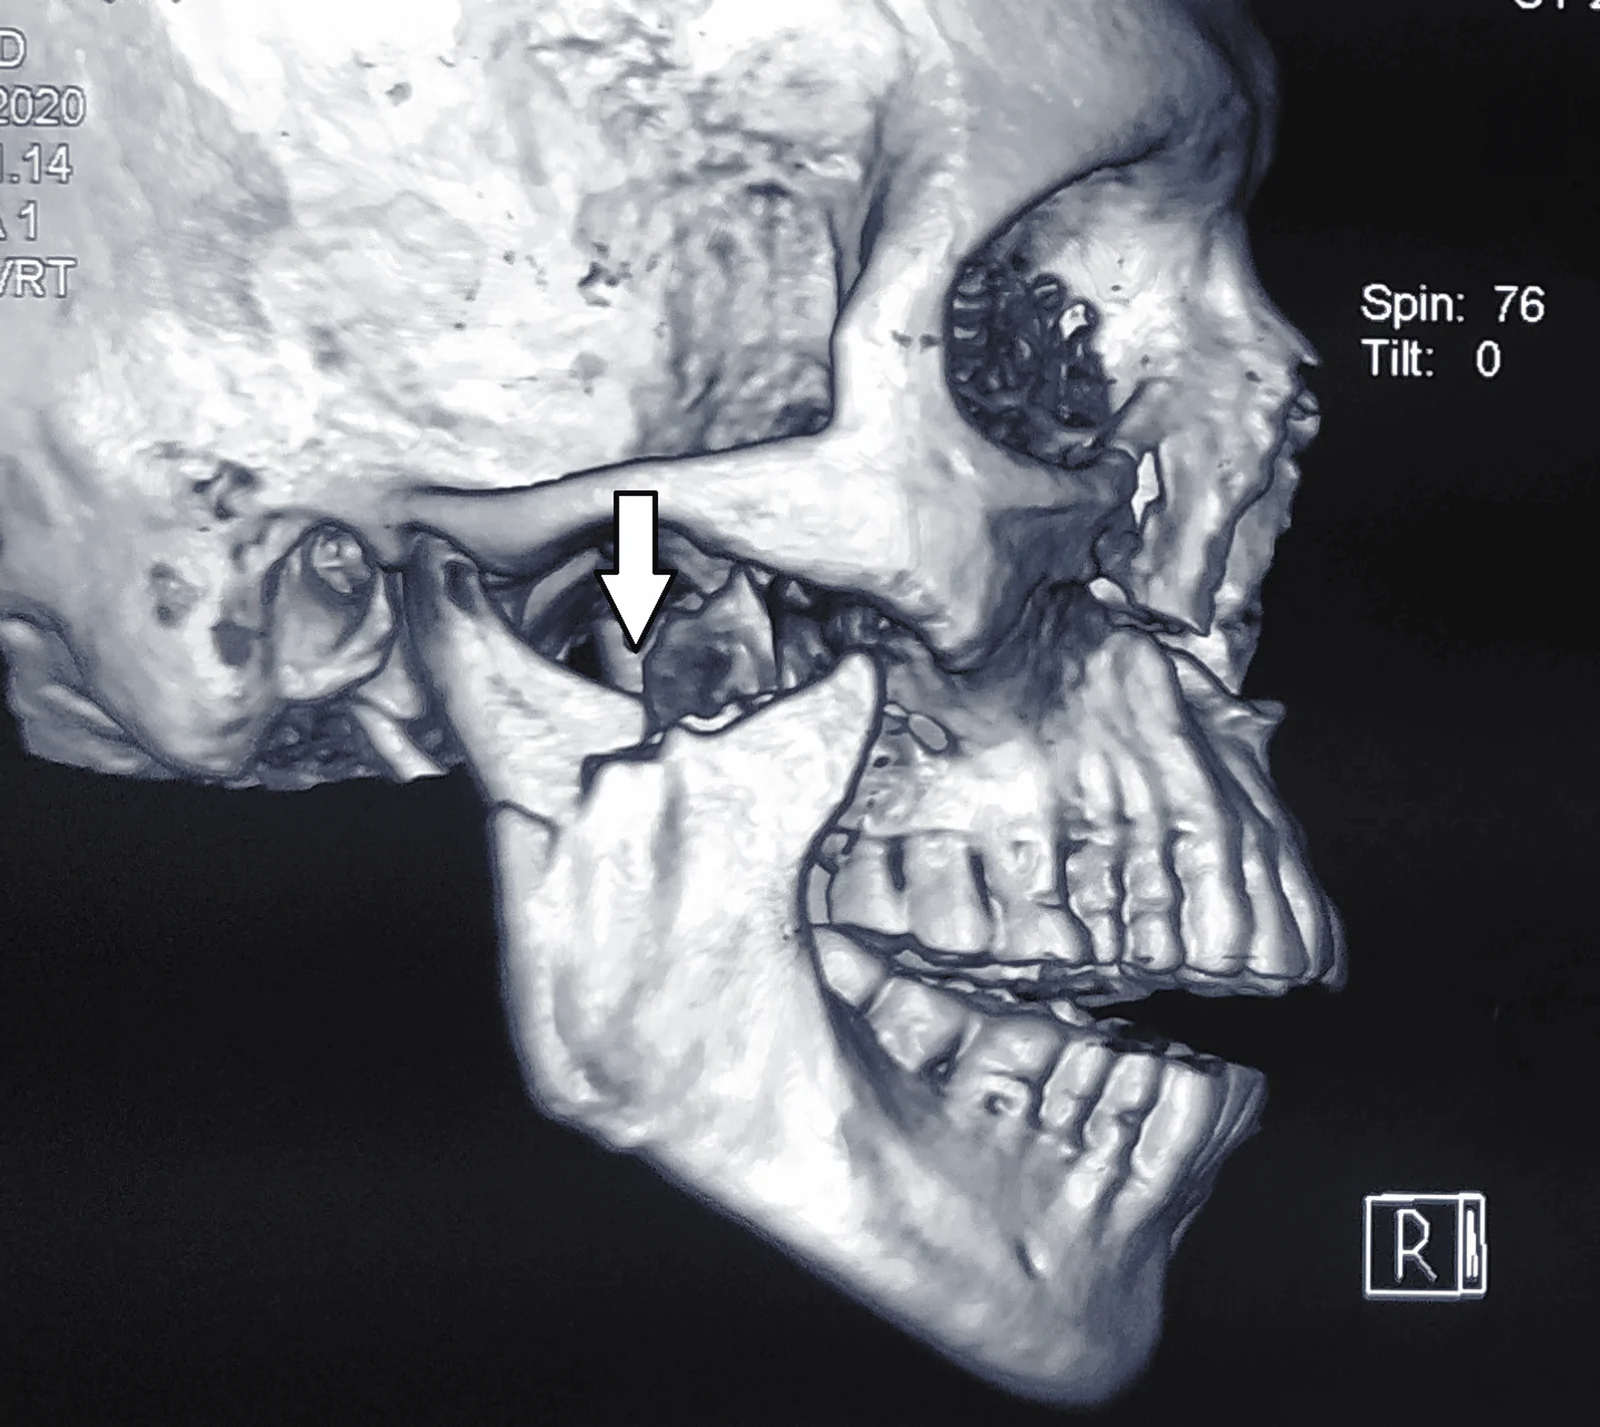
Three-dimensional computed tomography showing subcondylar fracture displacement depicted by the white arrow.
